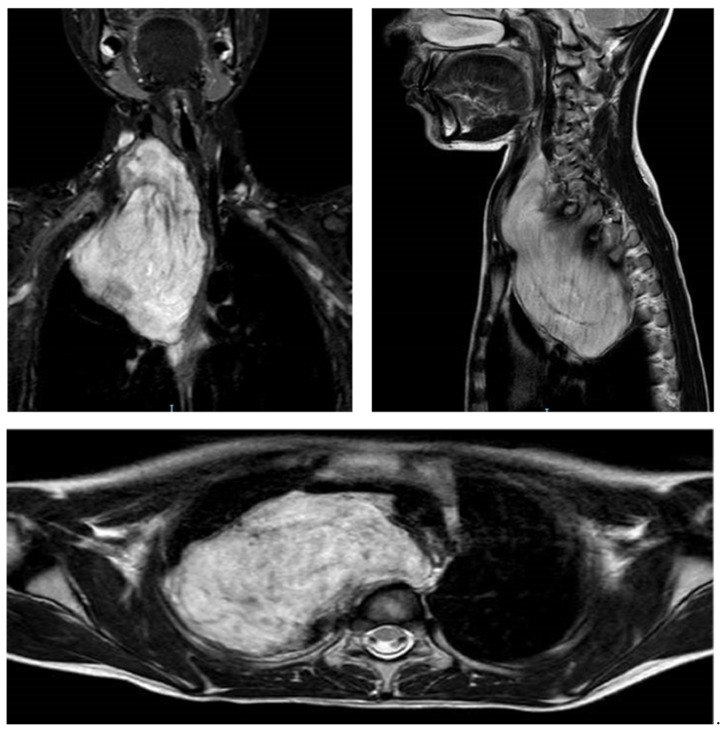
MRI scans of a patient affected by a cervicothoracic NB treated with CPT type B. The tumour causes the antero-lateral dislocation of the internal jugular vein, the right carotid artery, the trachea, and the bronchial biforcation. Posteriorly it penetrates into the epidural space (T1–T3), without compressing it. Inferiorly it extends toward the interscalen space along the brachial plexus. The subclavian and vertebral artery are in strict contact but not infiltrated by the tumour.
